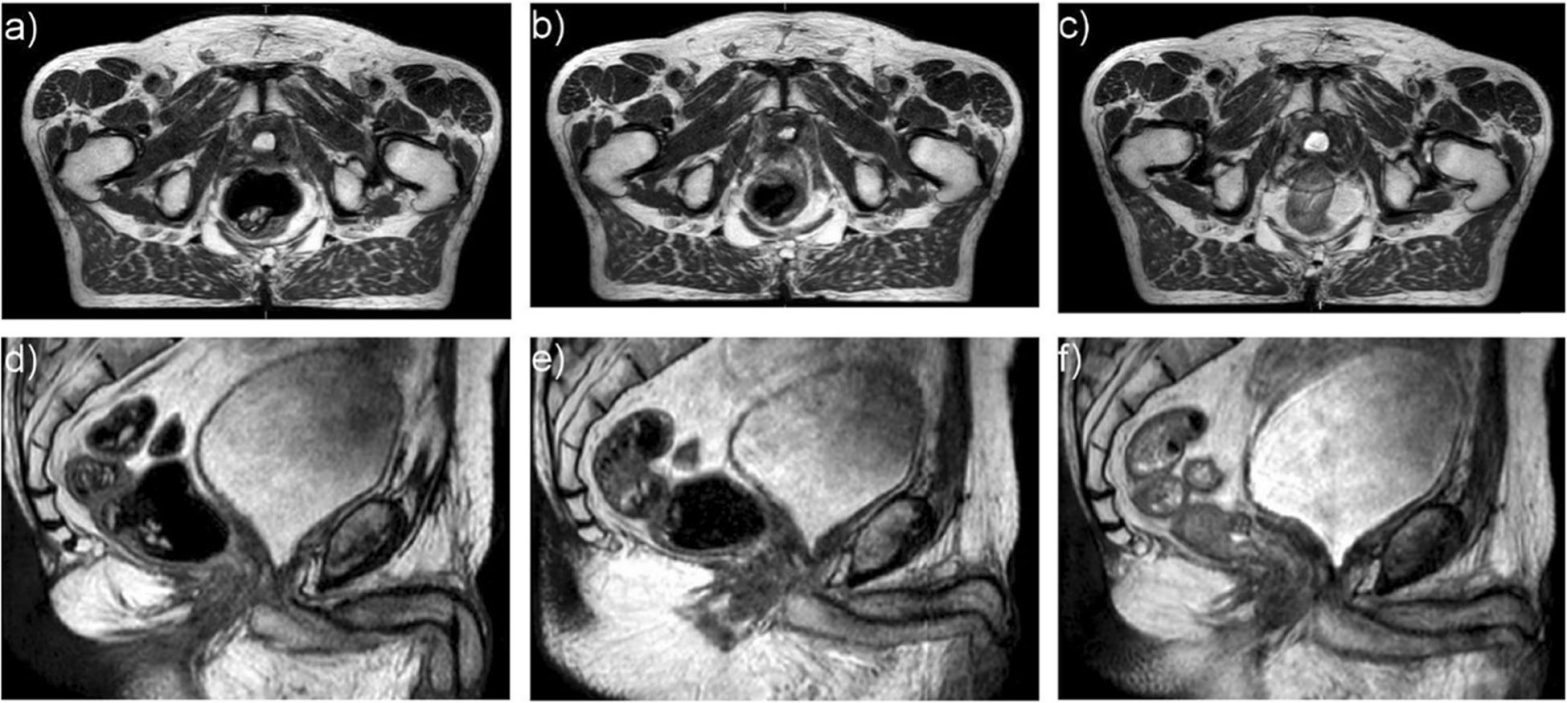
T2-weighted MR sequence of a postprostatectomy patient during MR-guided salvage radiotherapy at MR Unity (Elekta®). Axial (a-c) and the corresponding sagittal (d-f) views demonstrate different rectal volumes and different amounts of rectal gas during the course of fractionated radiotherapy
